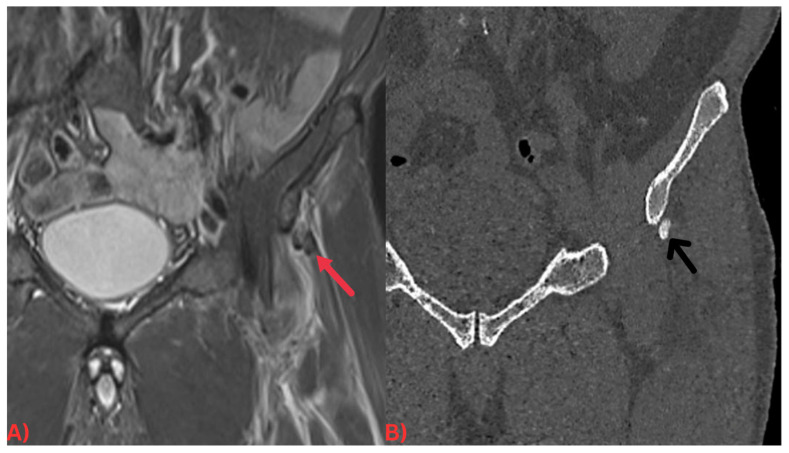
(A) Coronal short tau inversion recovery (STIR) MRI of the pelvis demonstrating an ill-defined low signal intensity focus at the origin of the left rectus femoris tendon (red arrow) with surrounding soft tissue edema. (B) Coronal CT image of the pelvis. Note how the calcification is more easily discernable on the CT image (black arrow).
